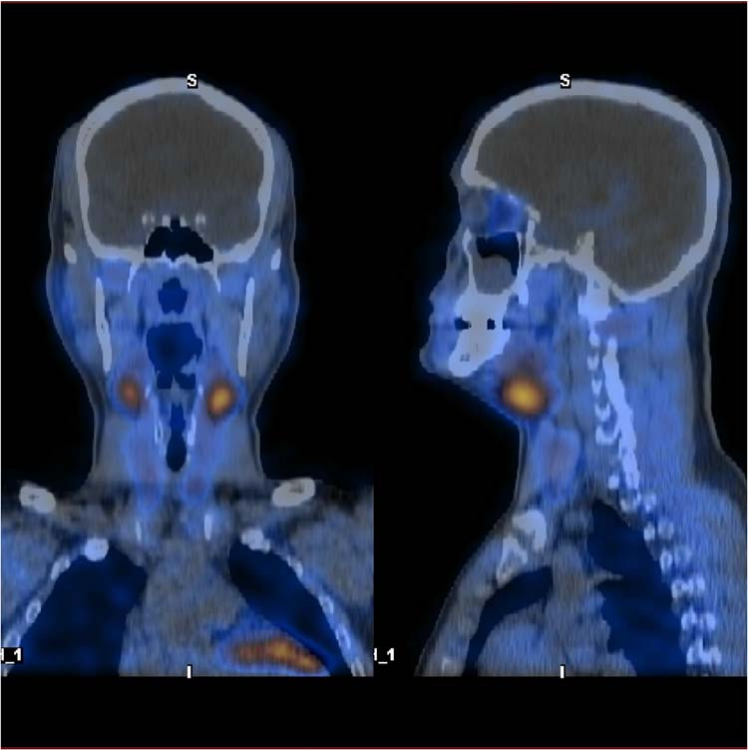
F-18 FCH PET/CT shows negative uptake.
